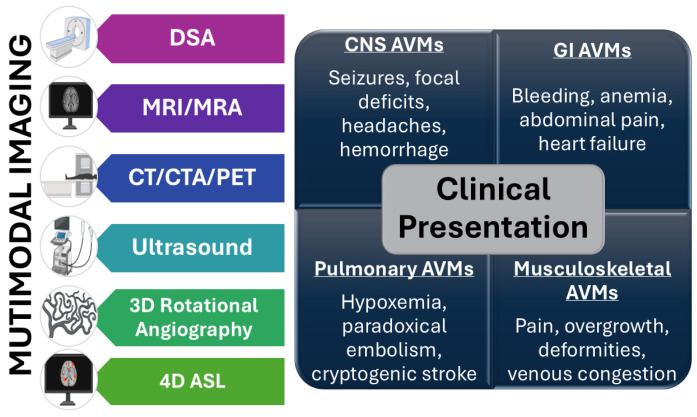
AVM diagnosis requires a tailored, patient-specific approach that integrates clinical evaluation with multimodal imaging, including digital subtraction angiography (DSA), magnetic resonance imaging and magnetic resonance angiography (MRI/MRA) with perfusion and susceptibility sequences, computed tomography angiography (CTA), Doppler ultrasound, 3D rotational angiography, arterial spin labeling (ASL), and 4D flow techniques refining hemodynamic assessment.
